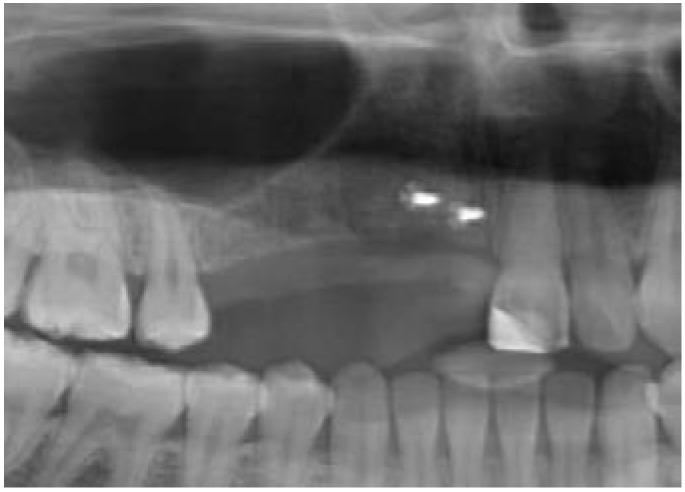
Panoramic radiographic investigation after fixation of the bone block. Surgeons: Dr. Stefan Roehling, Dr. Georges Ghazal (Image: Institute of Radiology, Kantonsspital Aarau, AG, Aarau, Switzerland).
